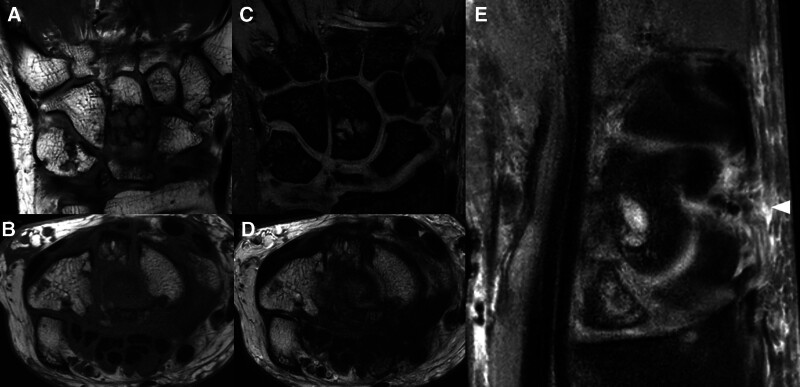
MRI. T1-weighted images on (A) the coronal and (B) axial view indicating the lunate bone as a low-intensity area. T2-weighted images on (C) the coronal and (D) axial view indicating the lunate bone as a partially high-intensity area. (E) The extensor tendon is not clear on sagittal T2-weighted images, which suggests rupture of the extensor tendon. MRI = magnetic resonance imaging.
